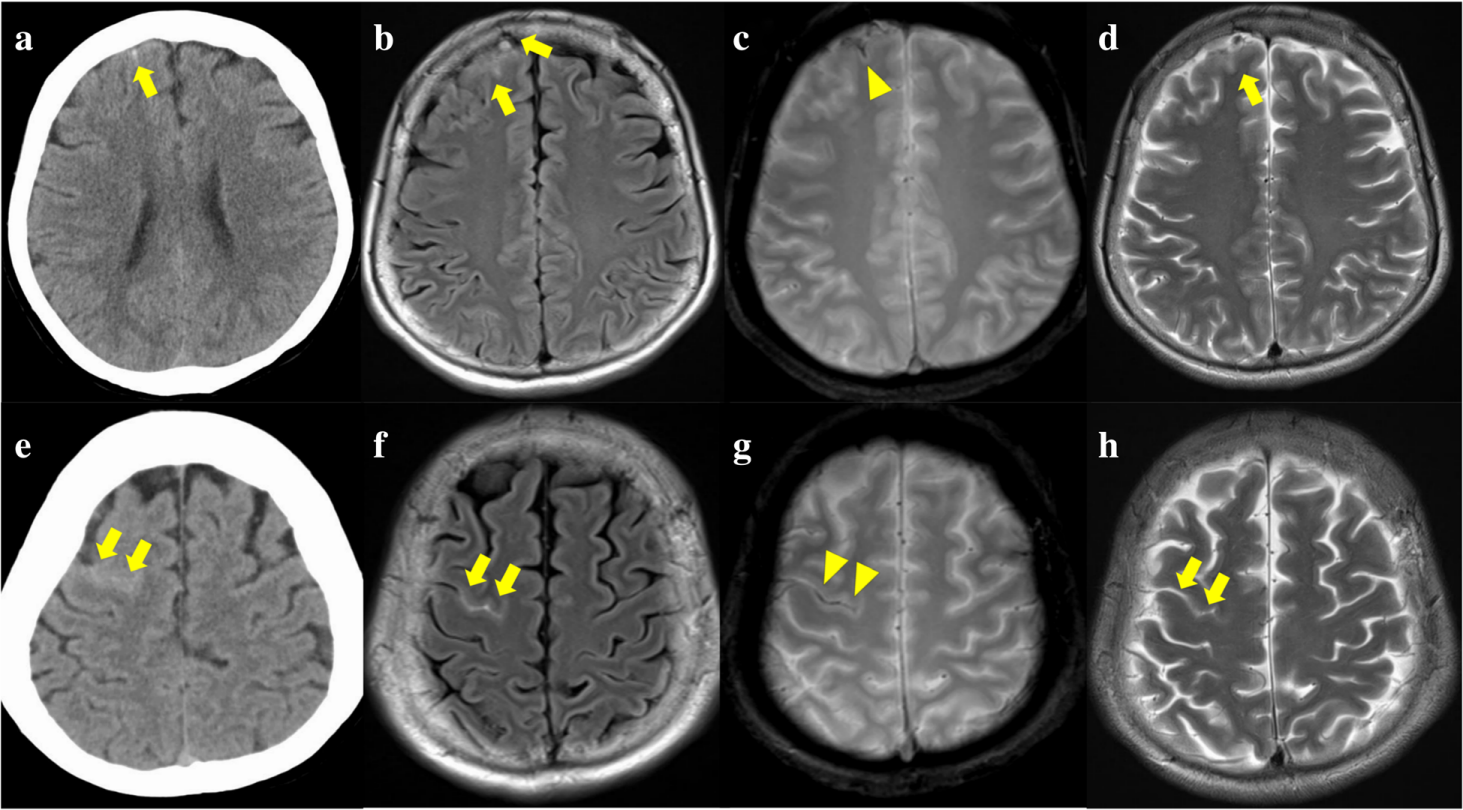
Head computed tomography and magnetic resonance imaging showed twice convexal subarachnoid hemorrhage on day1 of second admission (a-d), on day2 of second admission (e-h). Lesions have high density on computed tomography (a, e), a hyperintense signal on fluid-attenuated inversion recovery (b, e) and T2-weighted image (d, h) (arrow), and a hypointense signal on T2* susceptibility-weighted image (arrow head), which indicate not thrombosis but hemorrhage
